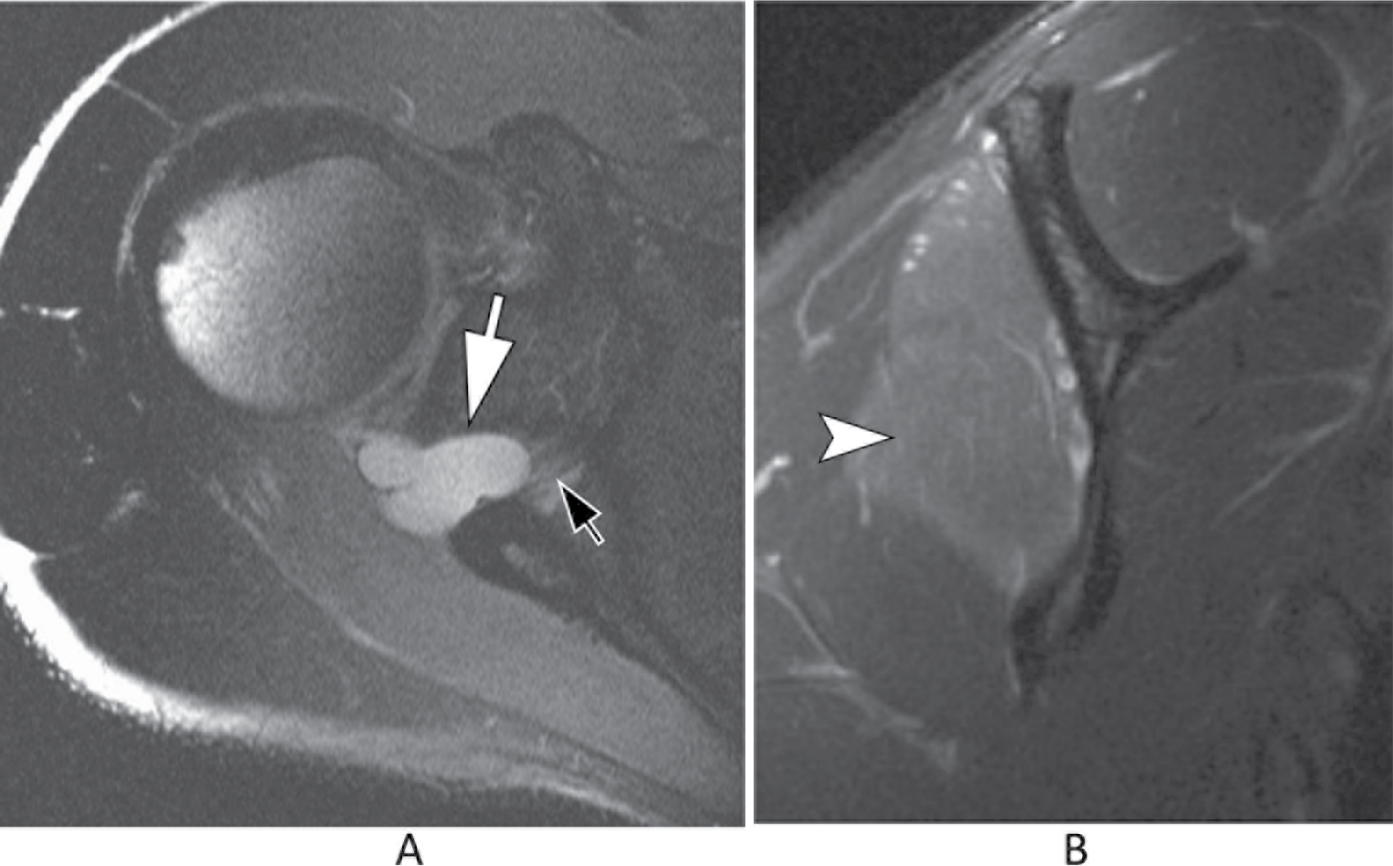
Suprascapular nerve compression. (A) Axial T2-weighted image of the shoulder. There is a paralabral cyst at the spinoglenoid notch (white arrow) with compression of the suprascapular nerve (black arrow). (B) Sagittal T2-weighted image with fat suppression shows denervation with edema at the infraspinatus muscle (arrowhead)
